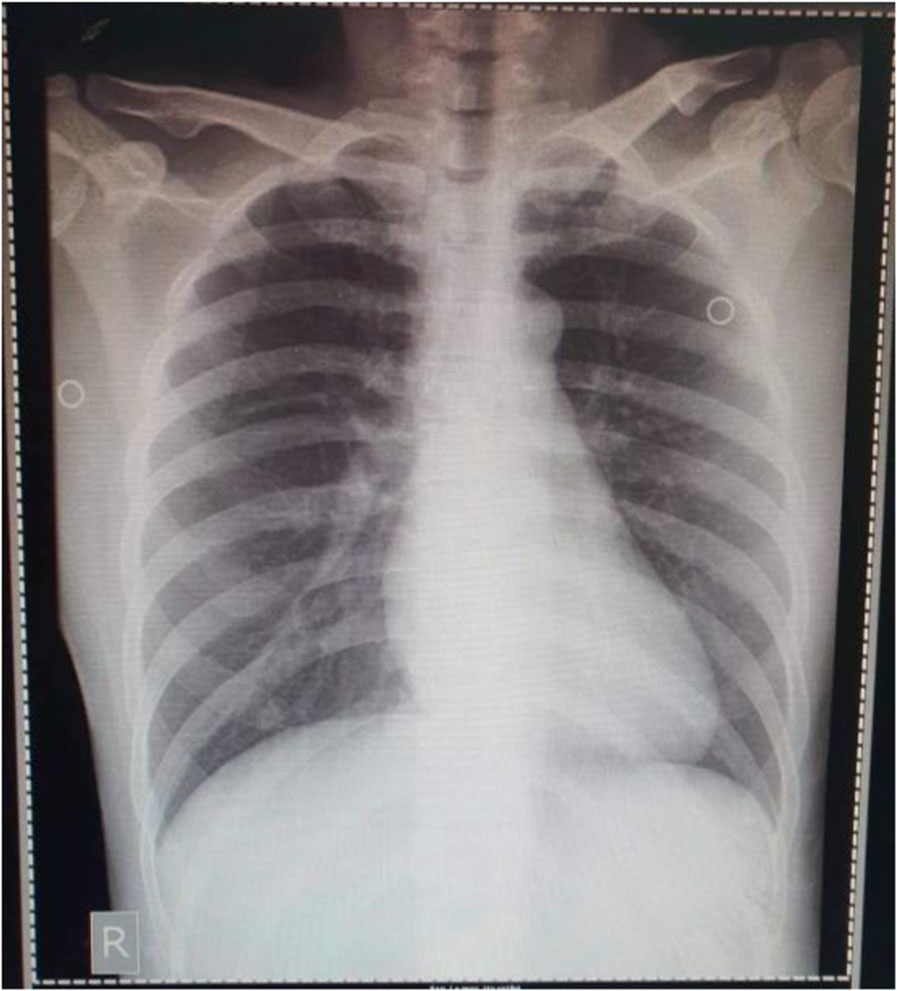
Posteroanterior chest radiograph of patient 1, 27 January 2020 (illness day 7). Unremarkable
